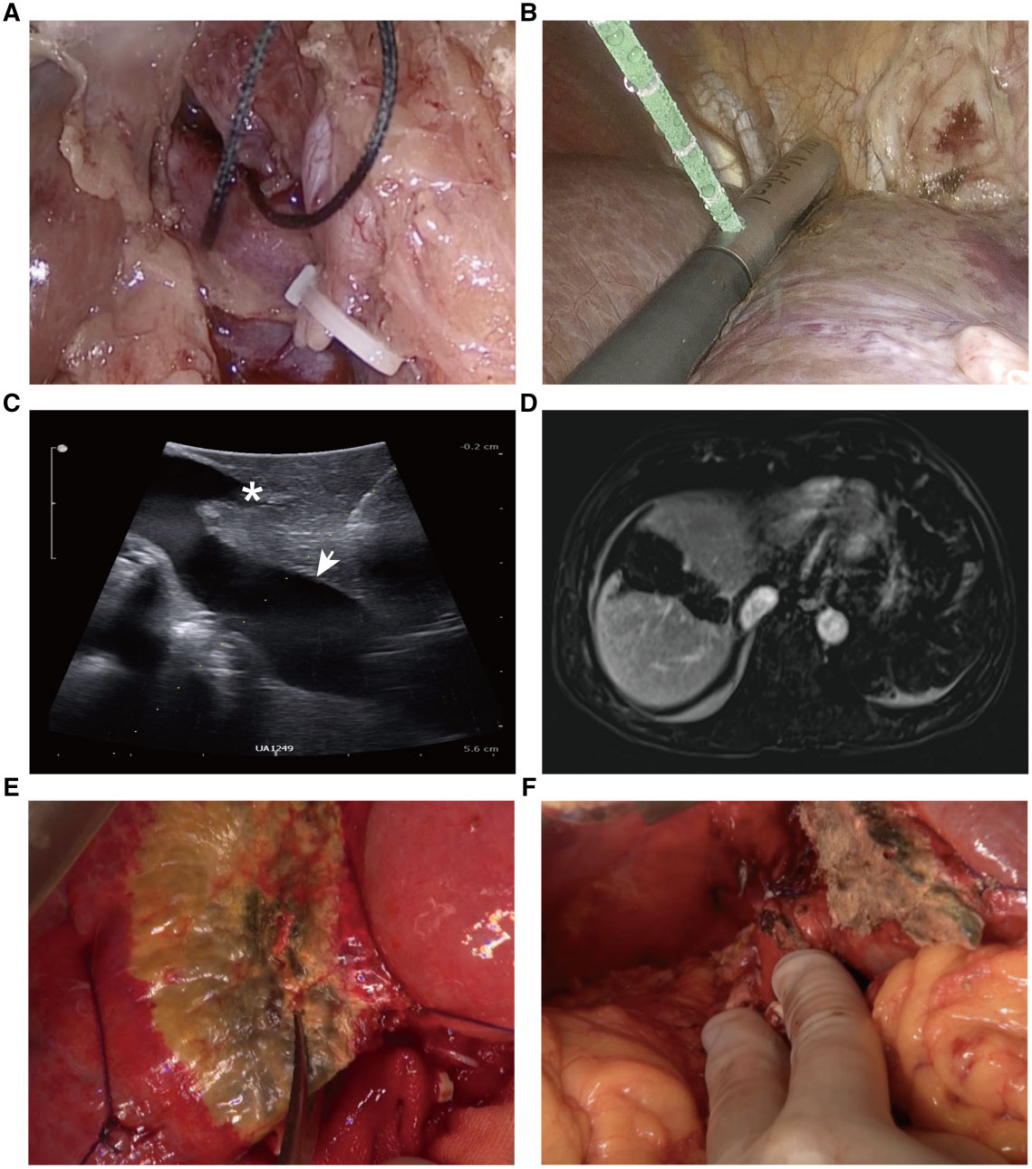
The procedure of laparoscopic microwave ablation and portal vein ligation for staged hepatectomy (LAPS). (A) Ligation of the right portal vein. (B) Microwave ablation guided by using ultrasound. (C) Microwave ablation zone (arrowhead: inferior vena cava; asterisk: middle hepatic vein). (D) Twelve days after stage-1 operation, MRI shows complete separation of the liver parenchyma. (E) Right hemi-hepatectomy was performed 15 days after the first operation. (F) Complete right hemihepatectomy.
